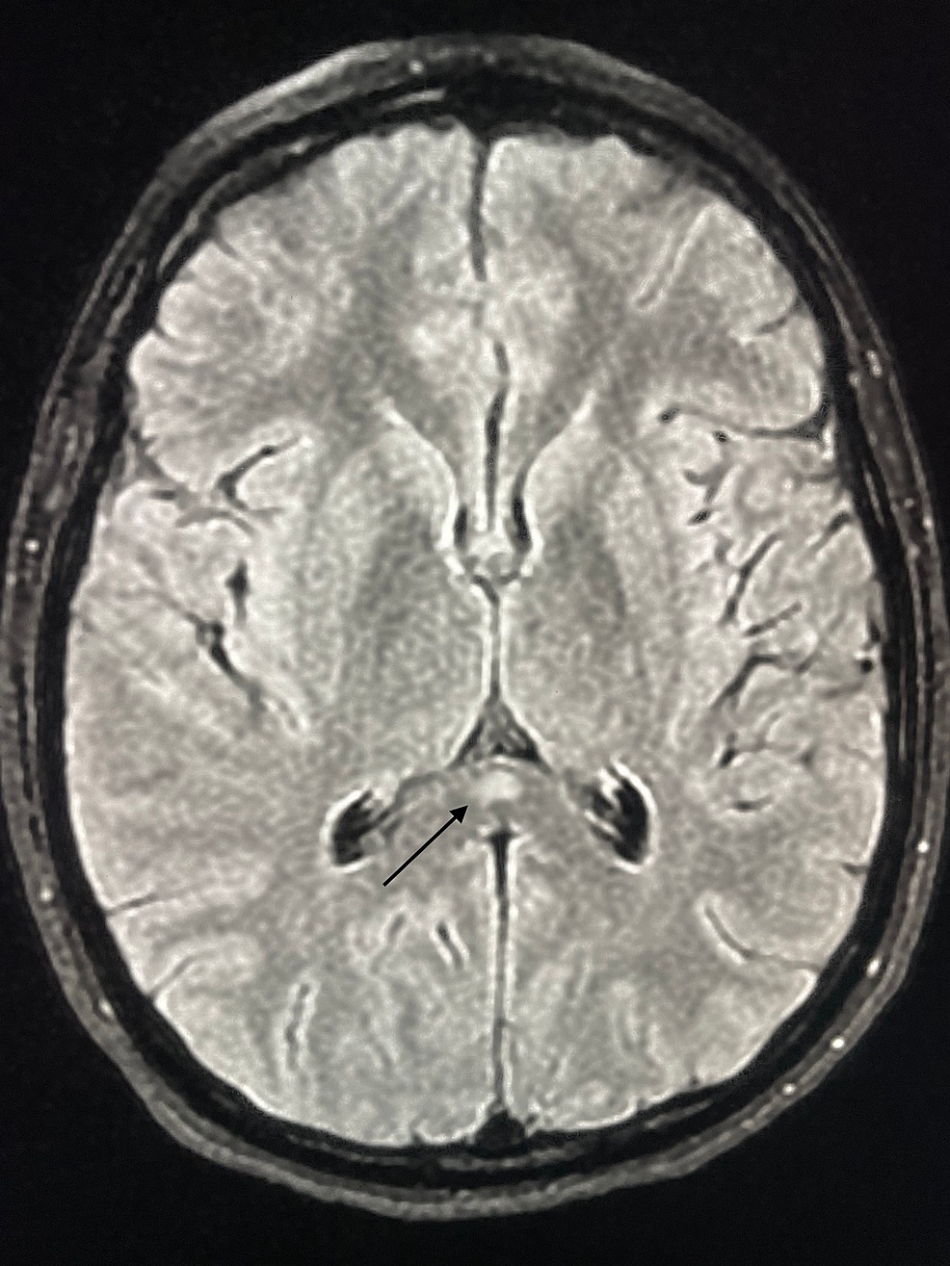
Brain magnetic resonance imaging performed before the antibiotic therapy (axial section, fluid-attenuated inversion recovery (FLAIR) imaging). FLAIR shows a focal, isolated, ovoid, hyperintense signal on the splenium of the corpus callosum (arrow).
